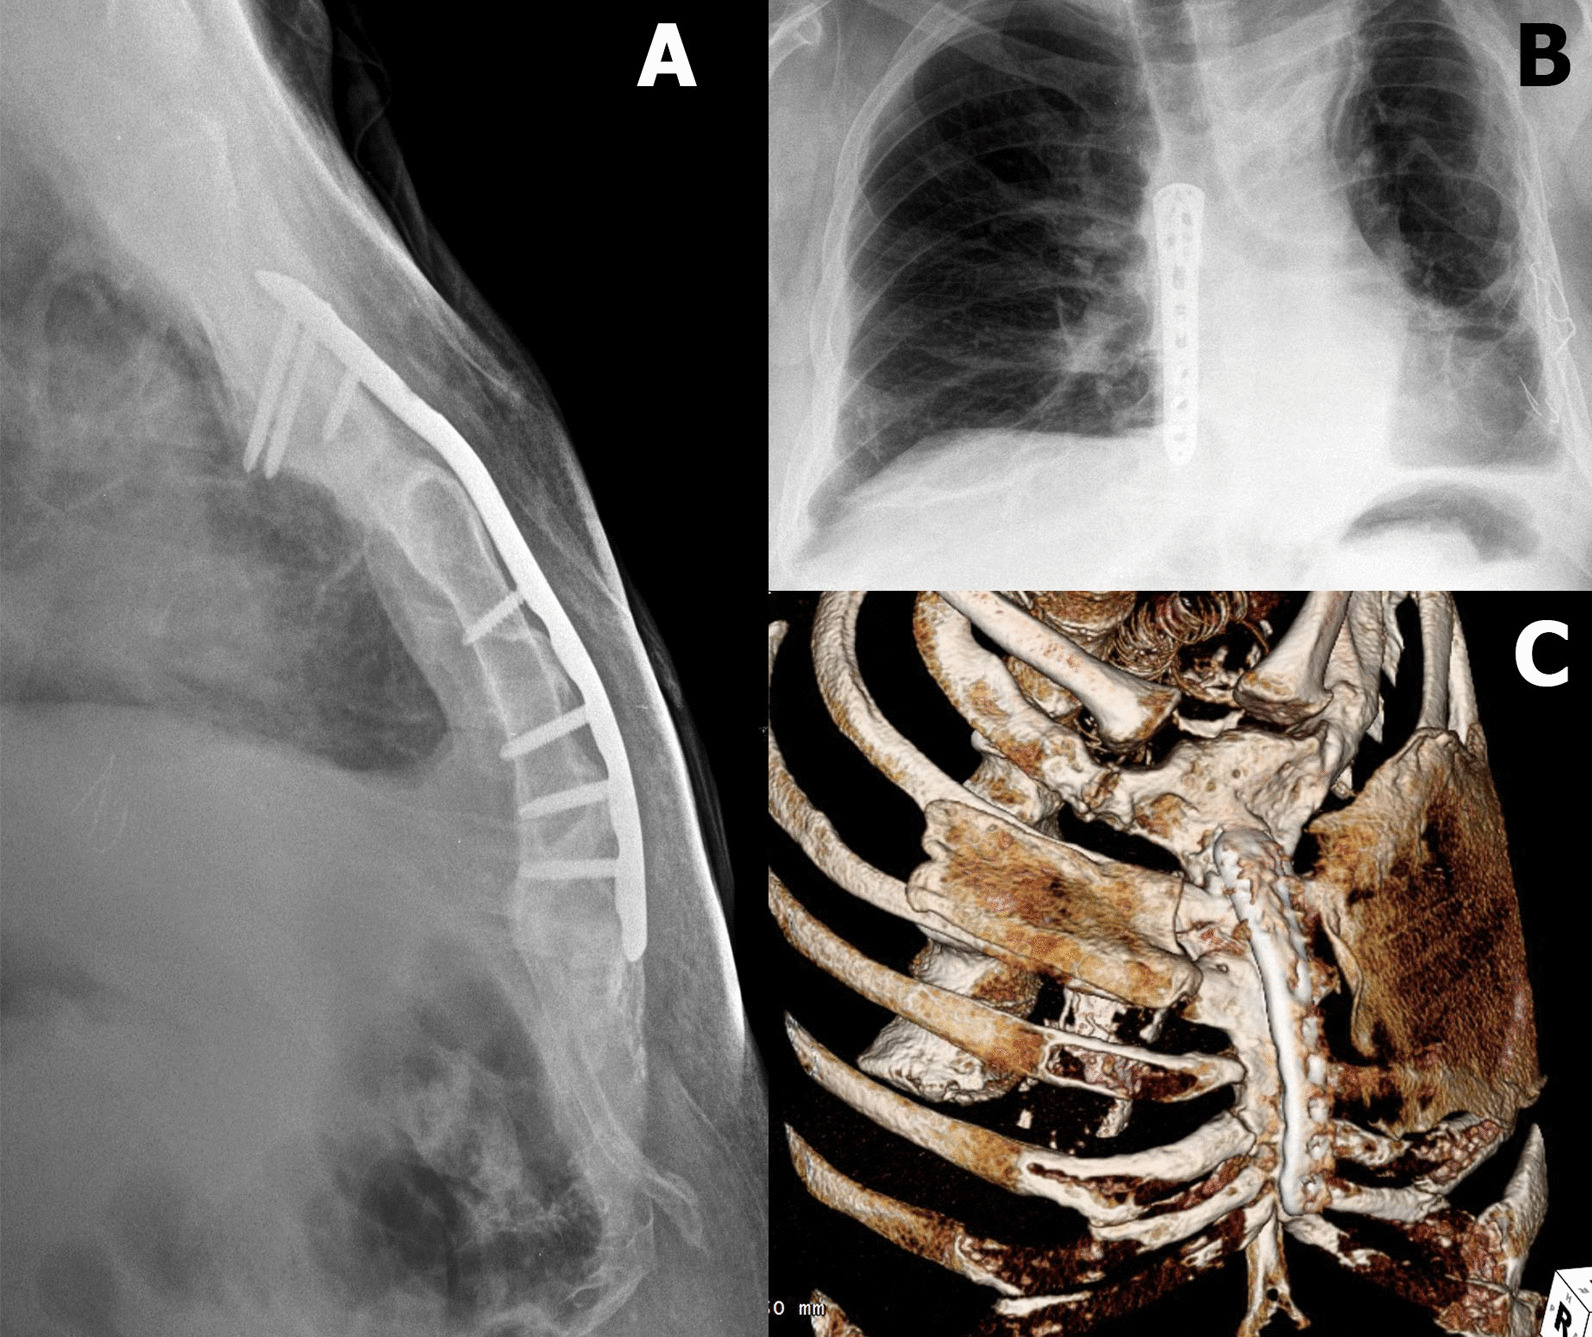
Chest X-ray. a lateral; b AP view. c 3D reconstruction of the CT 1 year postoperatively showing healing of the sternum and good Gore-Tex dual mesh membranes position
